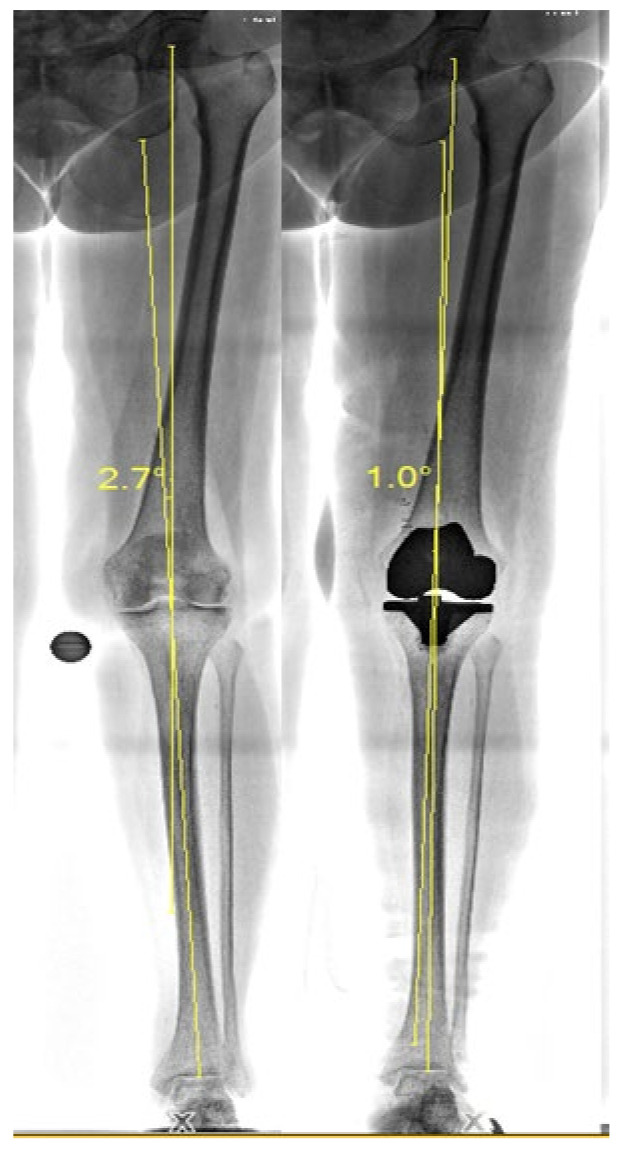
Pre- and postoperative long leg X-ray imaging with mechanical knee axis measurements.
